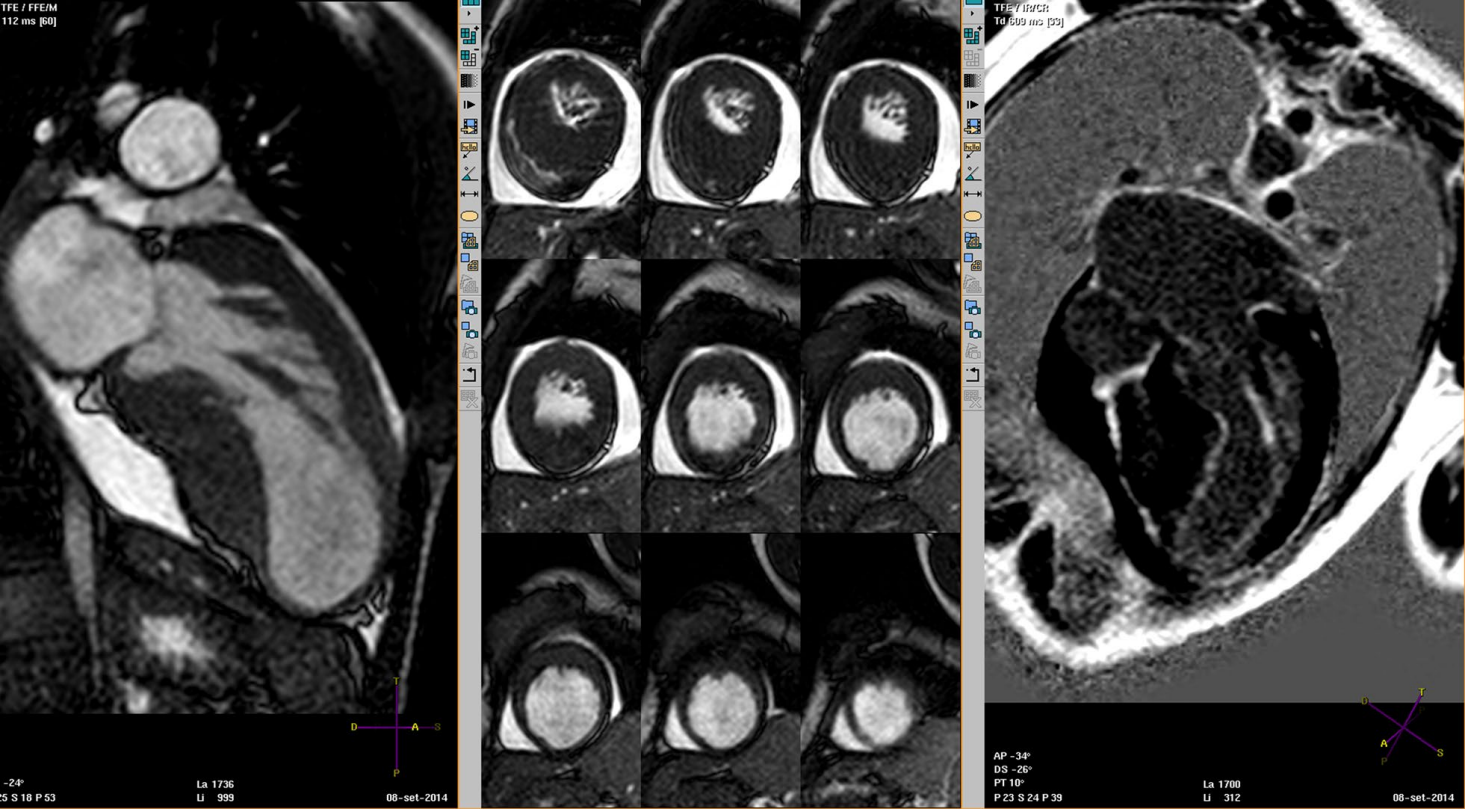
Heart MRI scans showing thinning and aneurysmatic dilation of apical myocardium, associated with transmural myocardial fibrosis
